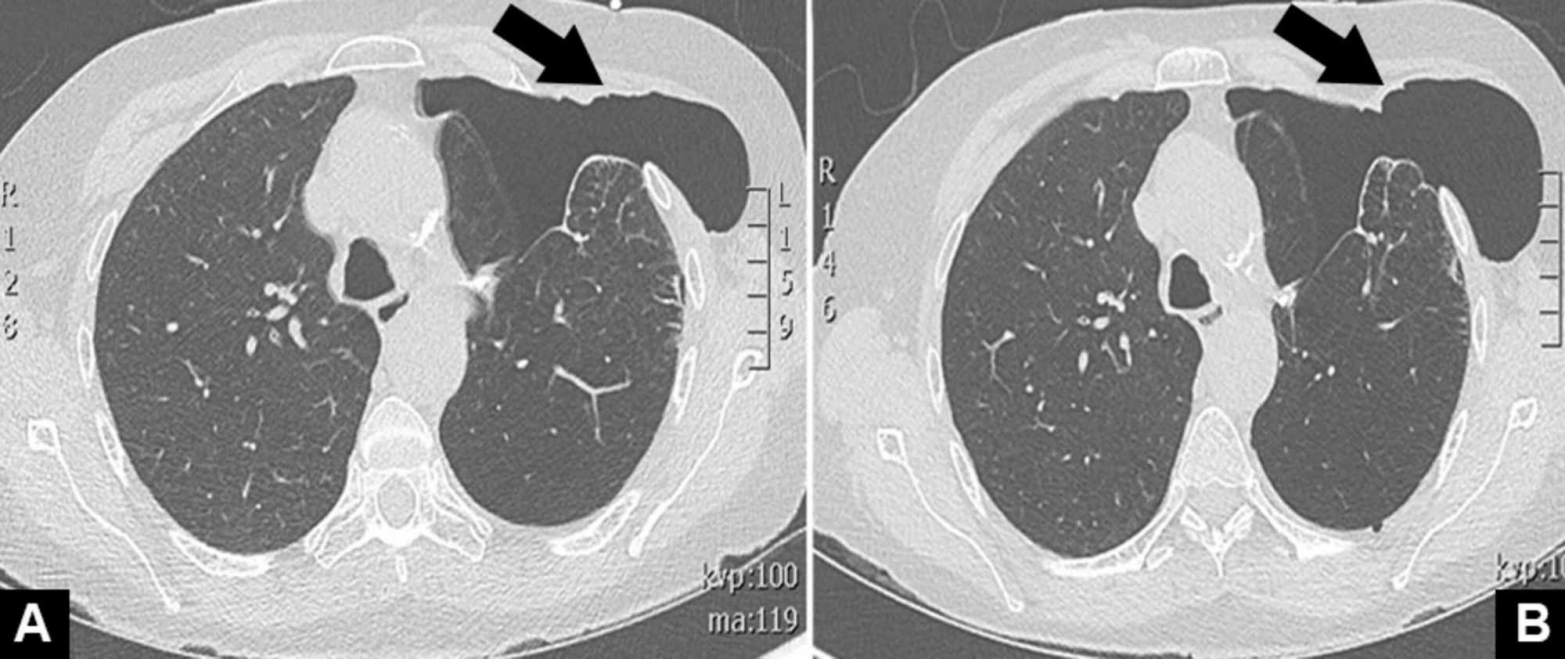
Non-contrast CT scan of the chest during inspiration (A) and exhalation (B) demonstrating left pneumothorax with herniation through the anterior chest wall.
